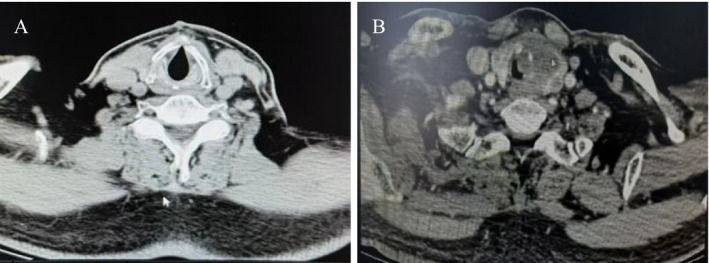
Neck CT in September 2023. (A) CT scan demonstrated no tumor detected in the glottic region. (B) CT demonstrating irregular mass occupying nearly 80% of the anterior circumference of the tracheal circumference.
